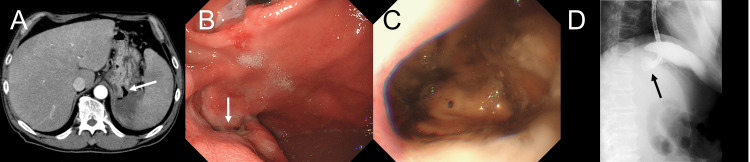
CT, EGD, and Gastric Fluoroscopy With a Gastrografin Injection Images. (A) Abdominal contrast-enhanced computed tomography (CT) shows the fistula (white arrow), which penetrates the dorsal wall of the gastric fundus and establishes a continuous connection with the gas-liquid cavity in the spleen. (B) Esophagogastroduodenoscopy (EGD) shows an opening of the fistula at the gastric fundus (white arrow). (C) A close-up EGD view showing the entire fistula opening. (D) Gastric fluoroscopy with a gastrografin injection shows the fistula between the stomach and spleen without any contrast leakage (black arrow).
